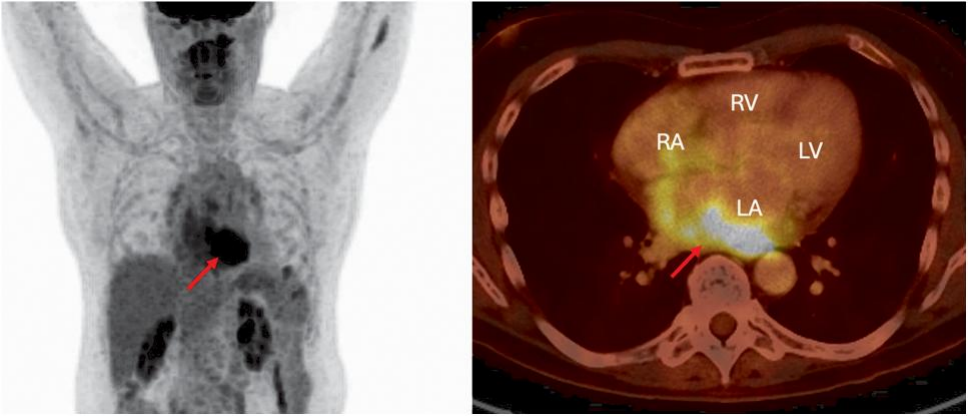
Positron emission tomography–computed tomography reveals a hypermetabolic cardiac mass (arrow) extending from the posterobasal wall of the left atrium to the ostium of the right pulmonary veins.
